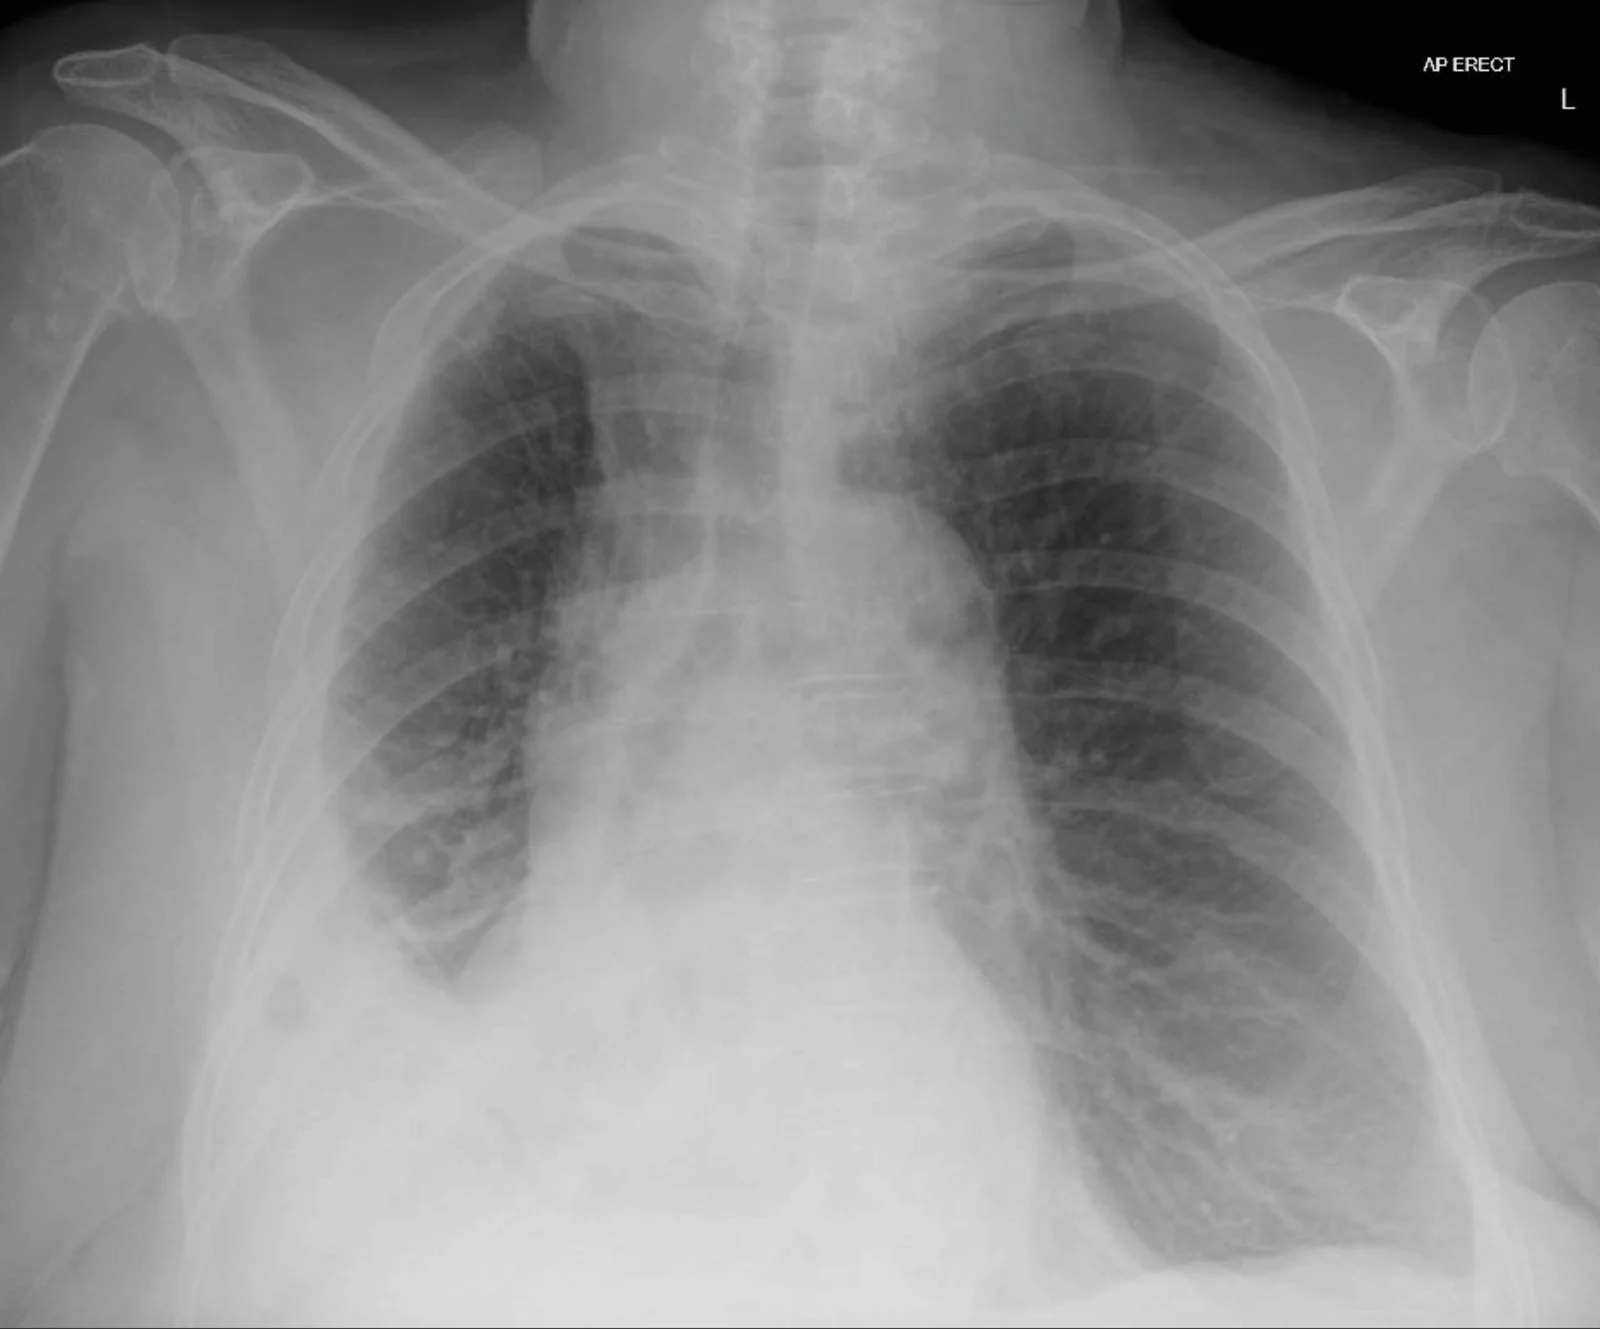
Chest radiograph showing right-sided lower-zone opacification, demonstrating a right-sided pleural collection representative of right-sided empyema thoracis.
